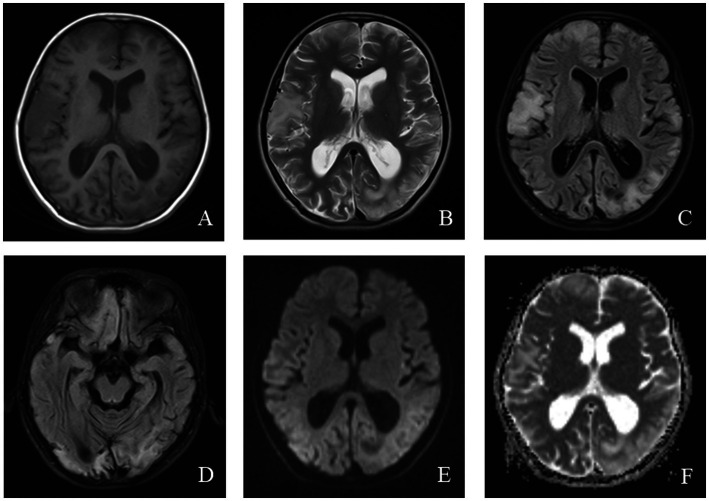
An 11-year-old female was diagnosed with MELAS syndrome due to intermittent convulsions for 3.5 years, which worsened over the last 7 days. Head MRI revealed: (A) Low signal intensity in the bilateral frontal, parietal lobes on axial T1WI. (B–D) On axial T2WI and T2/FLAIR, the brain tissue of the bilateral frontal parietal-occipital lobes was swollen, showing strip-like and gyrus-like high signal intensity. (E) DWI showed high signal intensity in the gyrus of the lesion area. (F) High signal intensity of the corresponding lesions was observed on the ADC.
